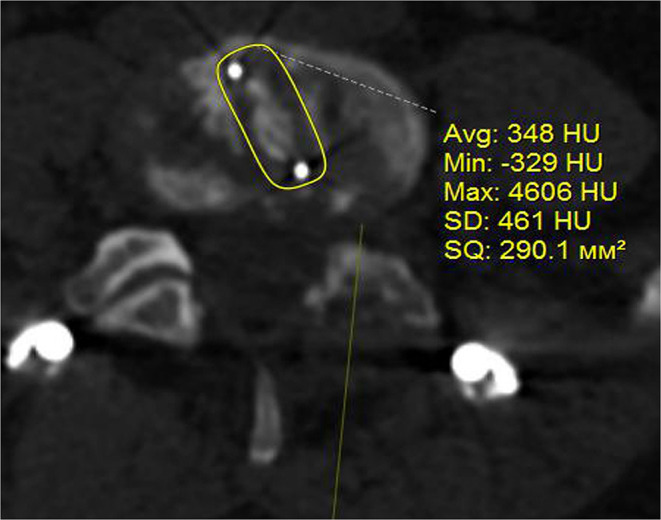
Cage used for TLIF, postoperative CT image in axial plane.
